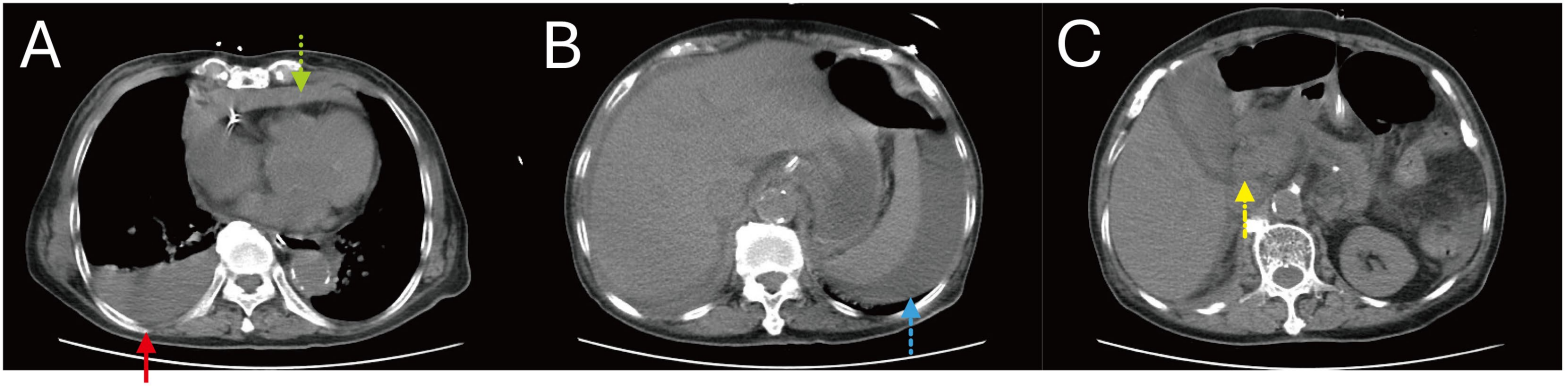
Patient 2, non-contrast abdominal CT at presentation. (A) Non-contrast abdominal CT at presentation showed a pericardial effusion (green dotted arrow), right pleural effusion (red arrow), (B) perisplenic ascites (blue dashed arrow), and (C) pancreatic head enlargement with peripancreatic inflammatory infiltration (yellow dash-dotted arrow).
